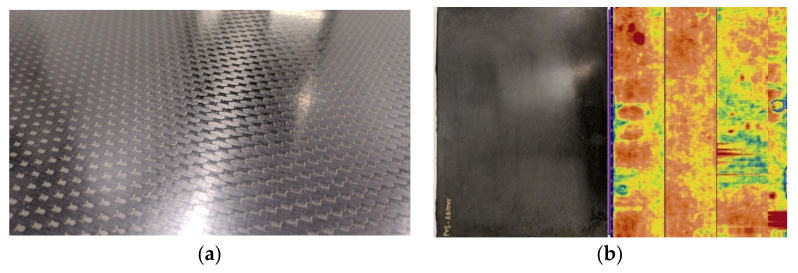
Preparation of AIR-3R test samples. (a) Visual inspection of the carbon fiber sheet manufactured inside the RTM molds. (b) Ultrasonic C-Scan inspection of the manufactured carbon fiber sheets prior to samples cutting.
